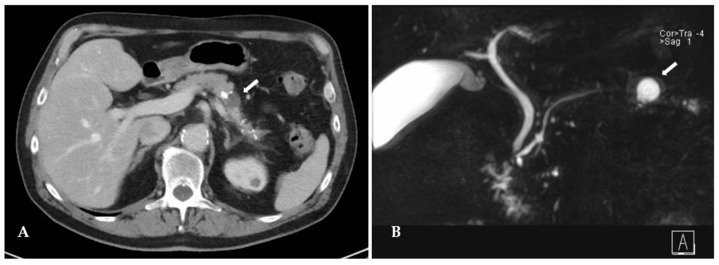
Preoperative CT (A) and MRCP (B) images of a cystic lesion (white arrow) in the pancreatic body.
